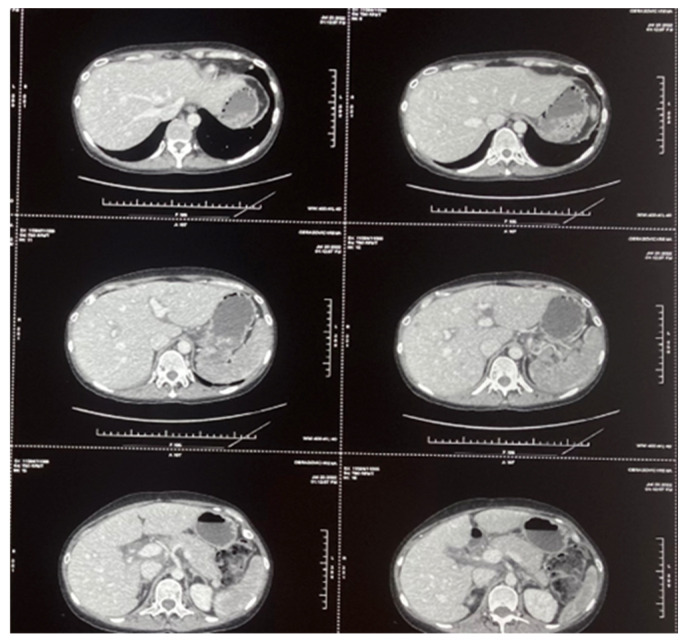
Multi-slice computed tomography (MSCT) revealed liver enlargement and extremely heterogeneous parenchyme with numerous lymphoma infiltrates.
